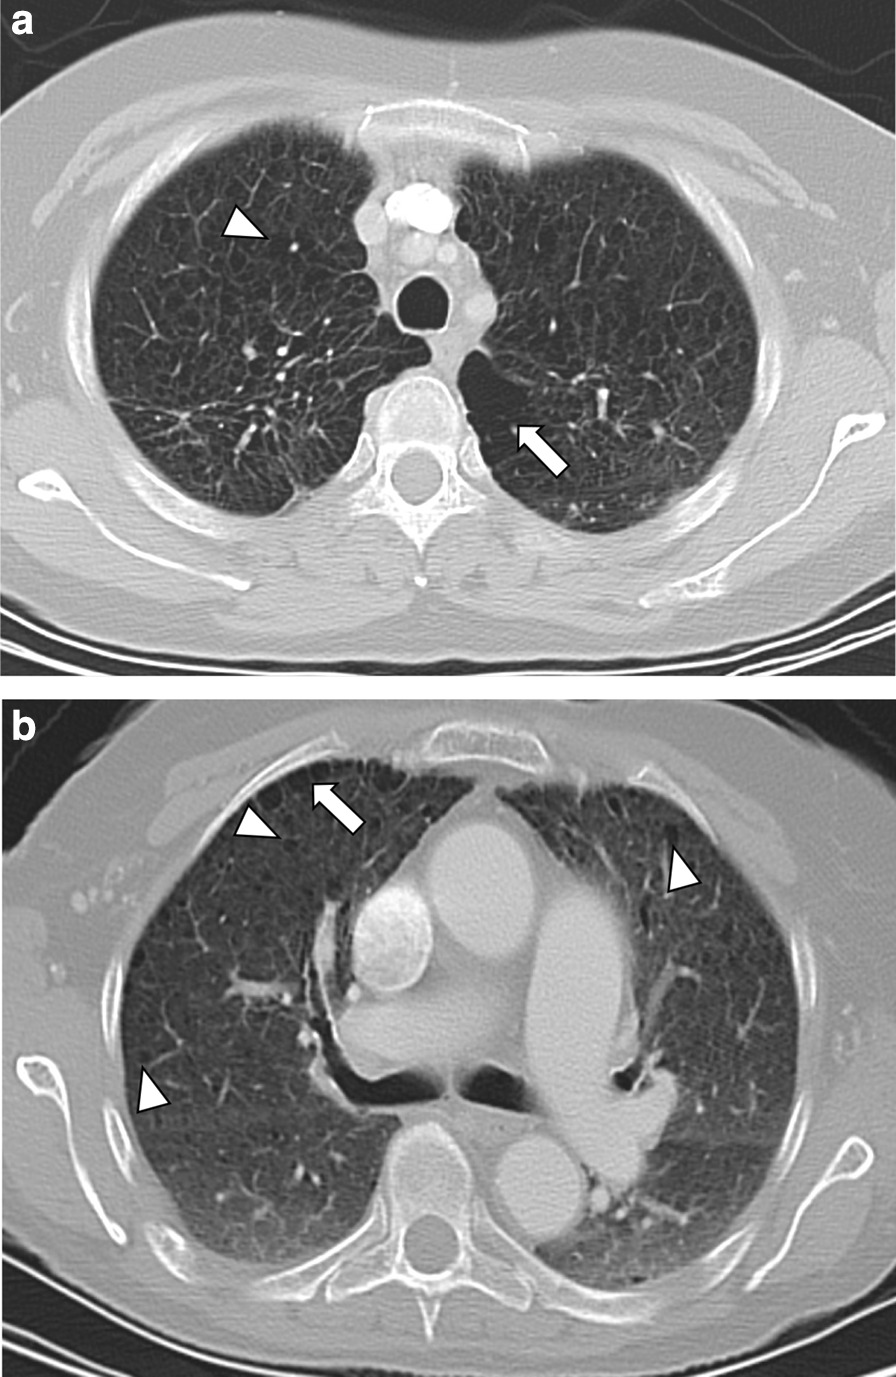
a Transversal CT image of a 52-year old female smoker with extensive centrilobular emphysema in both lung apices (white arrowheads) with bullae formation in the left apex (white arrow). b Transversal CT image of a 68-year old female non-smoker with a mild paraseptal emphysema (white arrow) and numerous pulmonary cysts in both upper lobes (white arrowheads)
